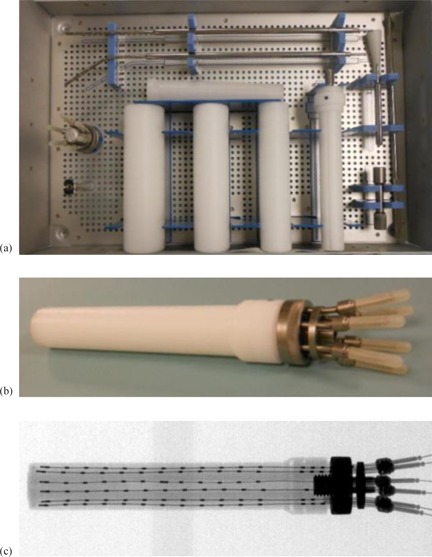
The Miami kit (a) in the sterilization box; photo of the Miami cylinder (b) with a 25 mm buildup cap; digital radiographic image (c) of the Miami cylinder with a 25 mm buildup cap and dummy wires inserted in all 6 peripheral channels.
